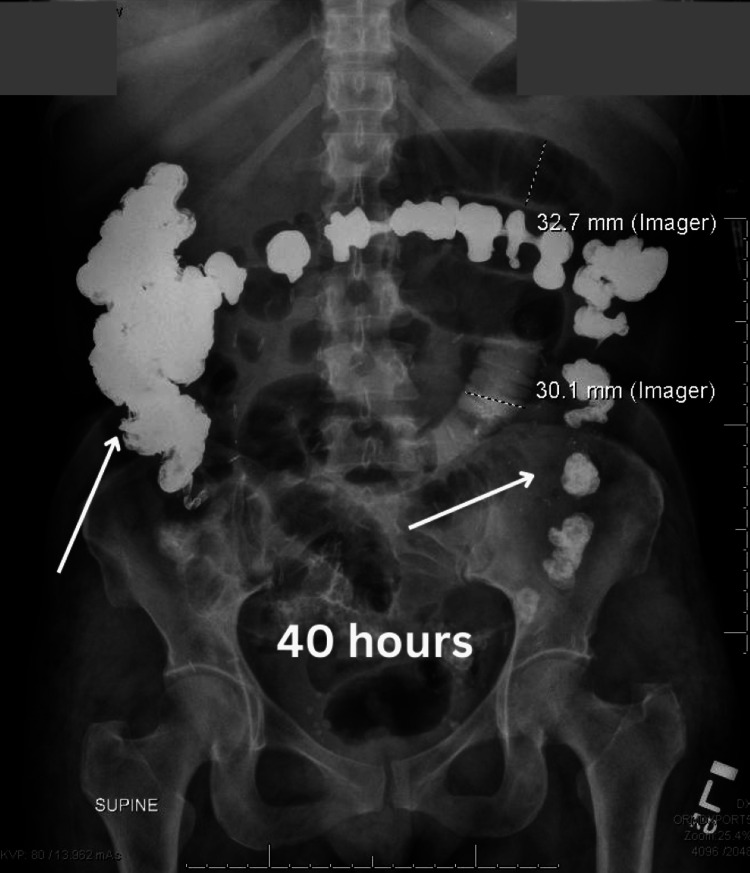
Abdominal radiograph demonstrating barium contrast located in the colon at 40 hours after small bowel follow through
